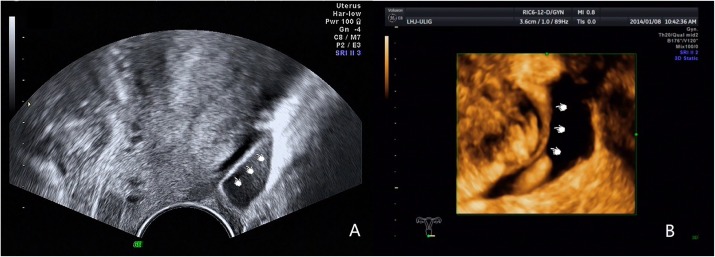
Images showing the normal USL imaged by 2D and 3D ultrasound in patients with fossa effusion. (A) Normal USL imaged by 2D ultrasound. (B) Normal USL imaged by 3D ultrasound.
